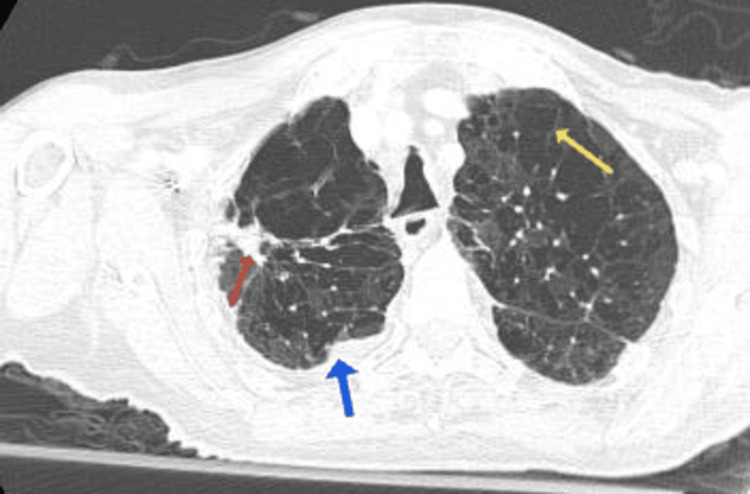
Chest CT demonstrating extensive emphysematous changes (yellow arrow), a right upper lobe spiculated lesion suspicious for malignancy (red arrow), and a right-sided pleural effusion (blue arrow)
